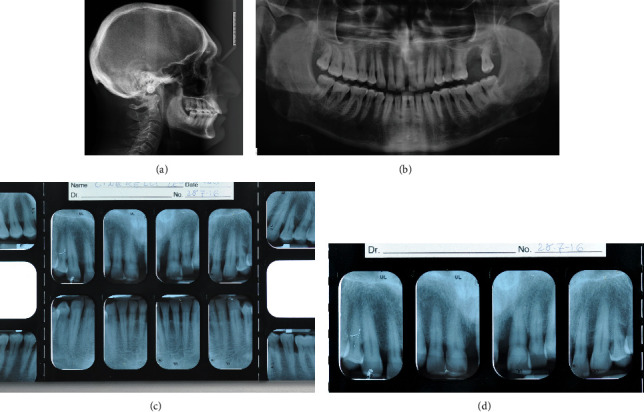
(a–d) Initial radiographic records.
